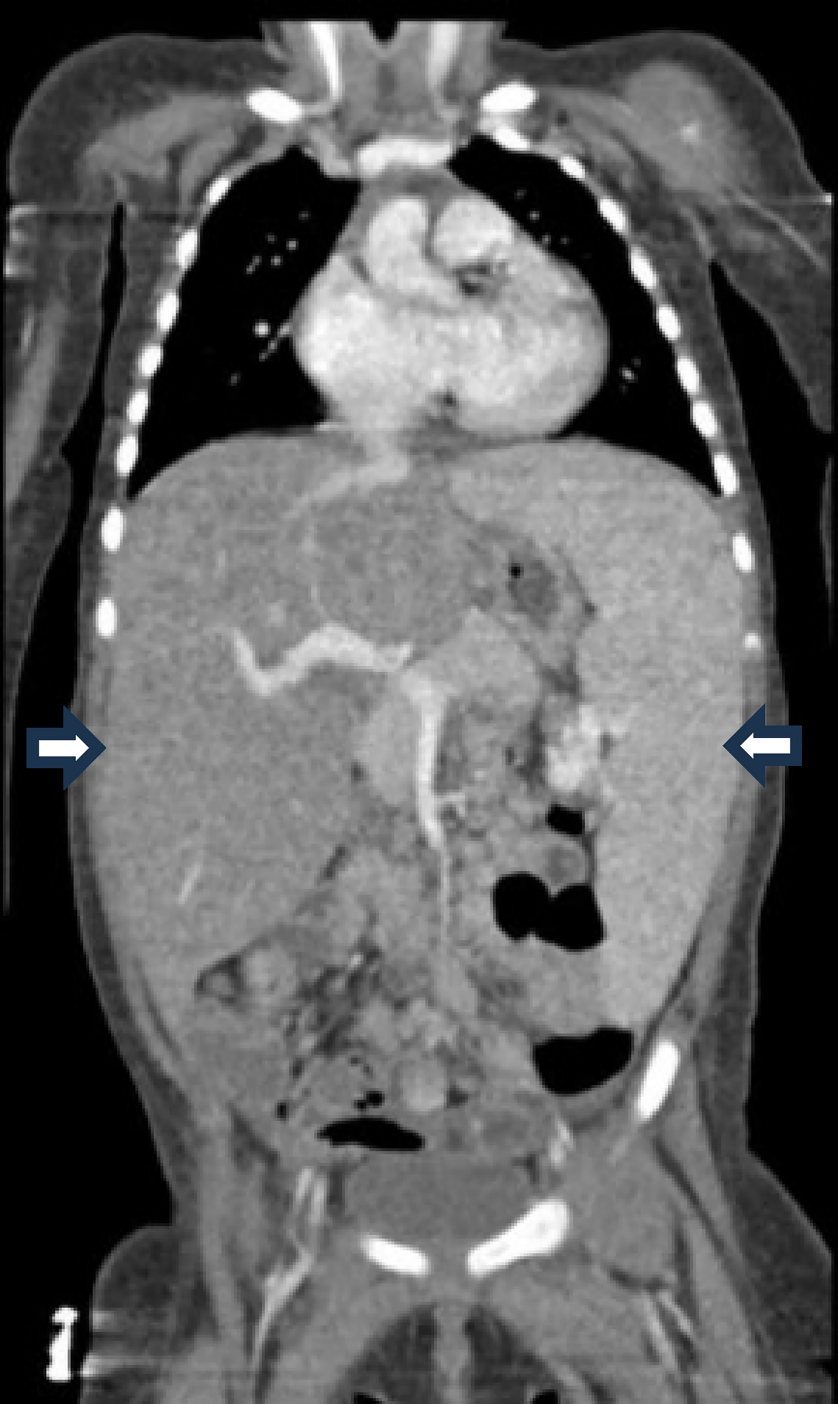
Hepatosplenomegaly visualized on abdominal computed tomography.
